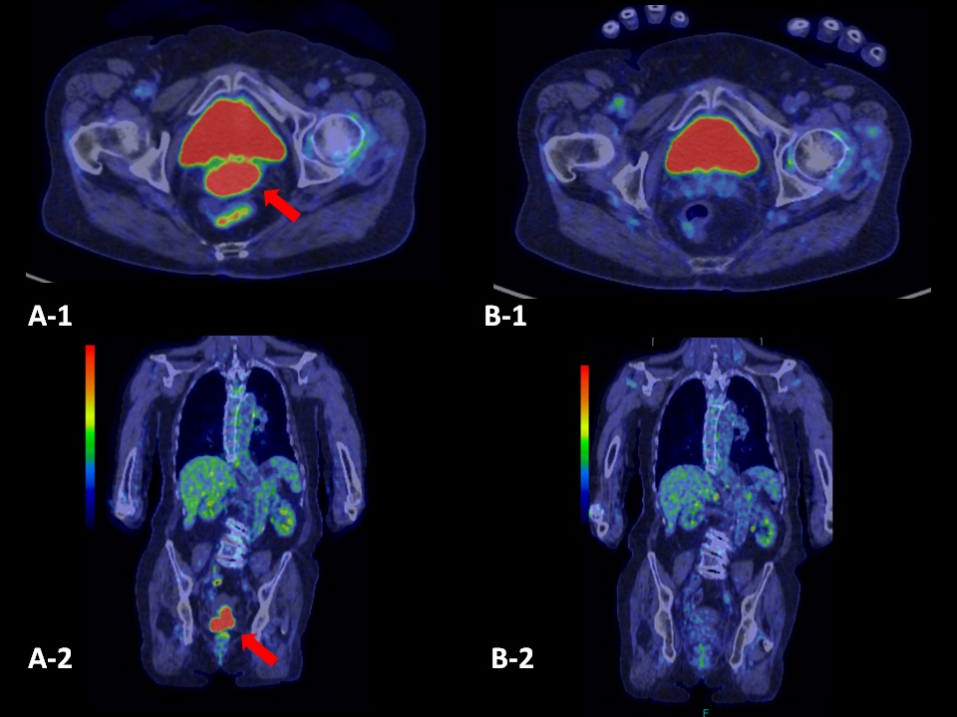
FDG (fluorodeoxyglucose)-PET of Case 1. A-1, 2: before SBRT. The FDG-uptake is shown in the cervix (the red arrows). B-1,2: 12 months after SBRT. The tumor has disappeared. (PET: positron emission tomography)
